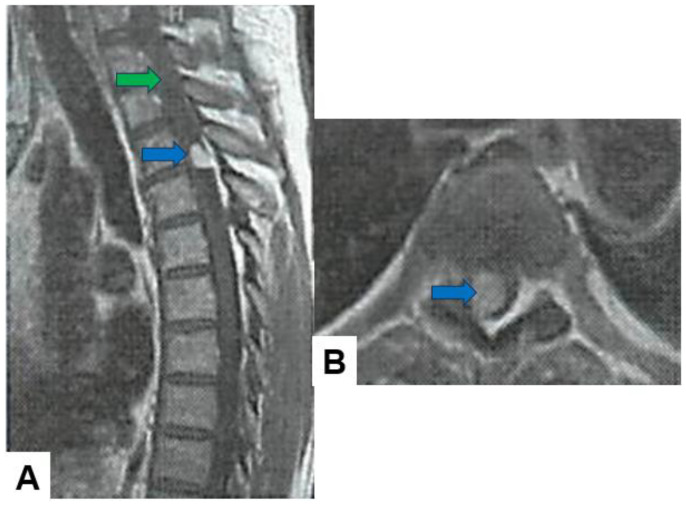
Fifty-four M, spinal hemangioblastoma, grade 1. (A) Enhanced T1-weighted midsagittal image; (B) enhanced T1-weighted axial image at T2/3. Blue arrows indicate tumor enhancement and green arrow shows syringomyelia.
